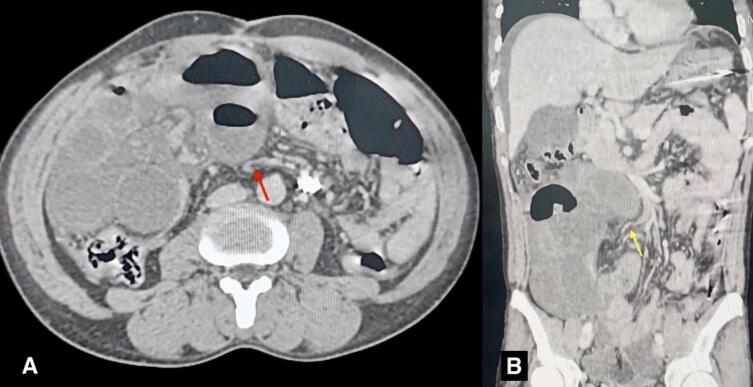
CECT scans axial (A) and coronal (B) sections showing a filling defect in the branch of the superior mesenteric vein (SMV) indicated by a red arrow in the axial section and a yellow arrow in the coronal section. CECT: Contrast-enhanced computed tomography. (For interpretation of the references to color in this figure legend, the reader is referred to the web version of this article.)
